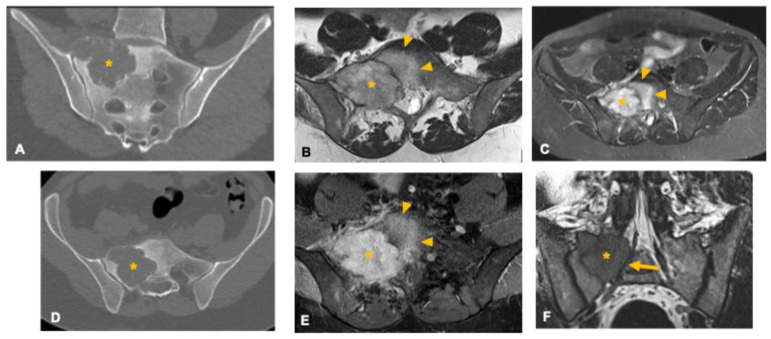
Osteoblastoma in a 33-year-old man. Coronal (A) and axial (D) CT images show a well-defined osteolytic lesion in the right sacral ala (asterisk) with lobulated contours, small internal calcifications, and surrounding sclerosis. The lesion demonstrates an expansile growth pattern with smooth scalloping of the anterior and posterior cortices, suggestive of slow progression. Axial T2-weighted (B), STIR (C), and post-contrast T1-weighted fat-suppressed (E) MR images depict an intermediate-to-high T2 signal lesion (asterisk) with diffuse enhancement and extensive perilesional edema (arrowheads), a hallmark imaging feature of osteoblastoma. Coronal volumetric T2-weighted image (F) demonstrates foraminal narrowing and radicular compression (arrow) caused by the expansile component.
